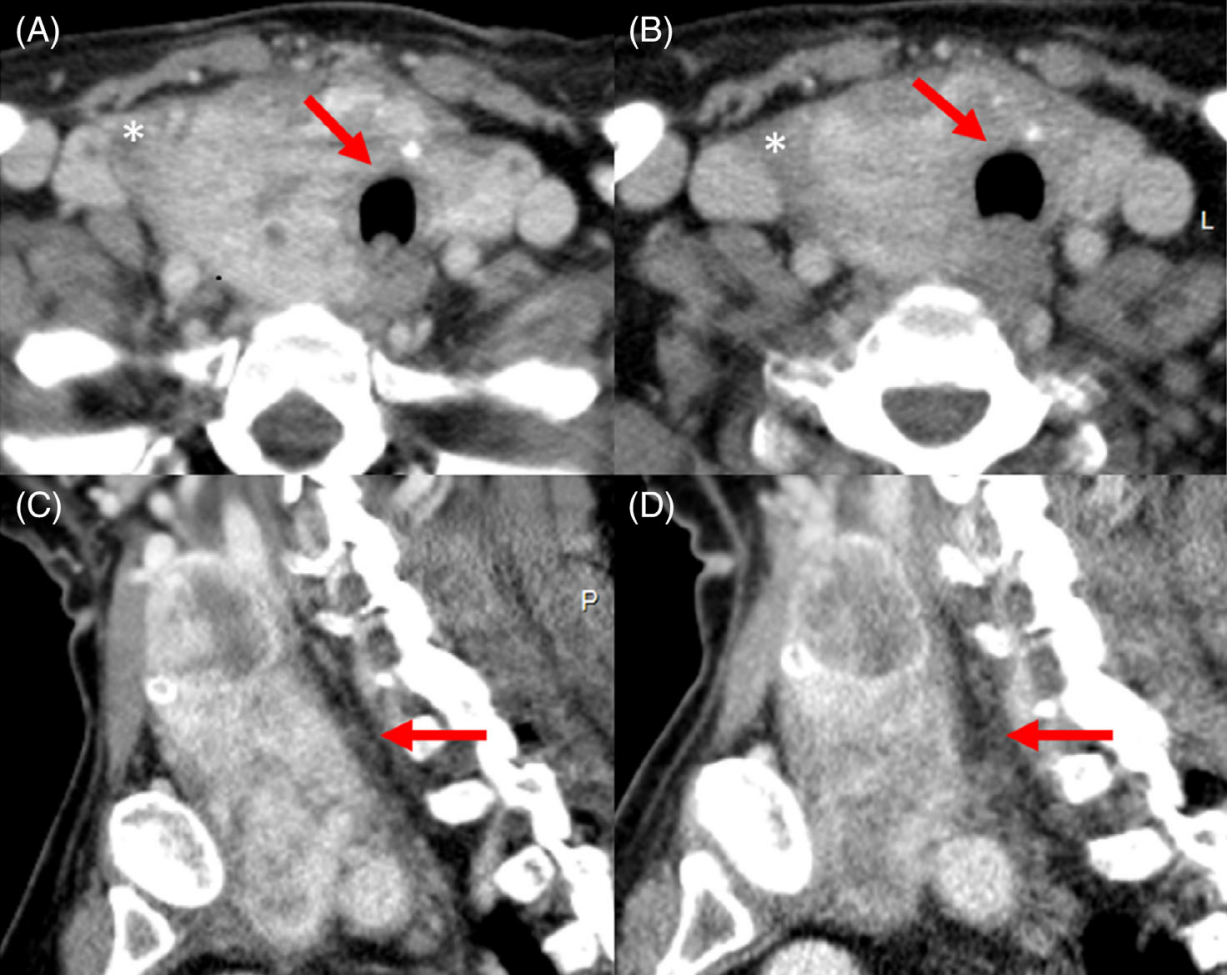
Axial and sagittal images from the CT examinations of the neck with contrast performed before and after Lenvatinib therapy. Axial (A) and sagittal (C) images before Lenvantinib treatment. Axial (B) and sagittal (D) images after Lenvatinib therapy. The axial images (A) and (B) demonstrate decreased mass effect on the trachea with widening of its lumen (arrows). Similar changes are noted on the right internal jugular vein (asterix). On the sagittal images (C) and (D), there is an overall subtle decrease in the size of the mass with decrease in the fullness of its posterior contour and increase in the fat posterior to the mass (arrow)
